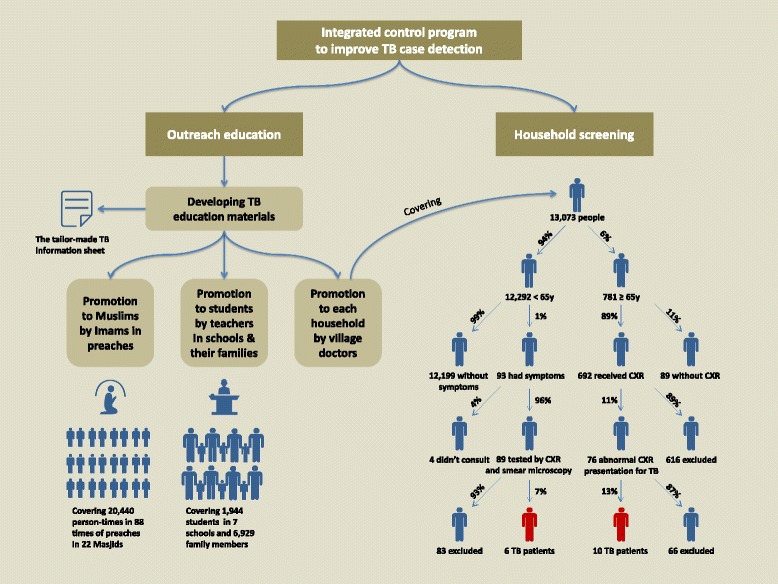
The conceptual framework and operational results of pilot interventions to improve case detection in underdeveloped multi-ethnic regions in China, 2014 – 2015 (TB: tuberculosis, 65 y: 65 years old, CXR: chest X-ray)
